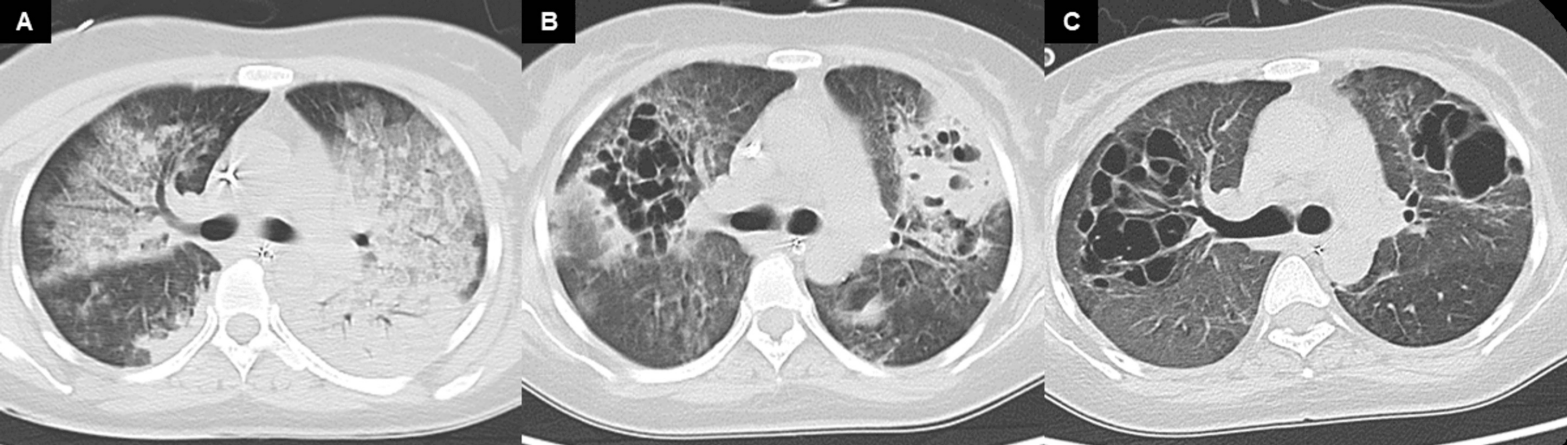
Changes in chest CT findings (A) On admission, CT scans revealed consolidation in the left lower lobe and bilateral GGO. (B) By the time of ECMO weaning, the GGO had resolved, but bilateral cavitary lesions from alveolar destruction and localized consolidation in the left lung were observed. (C) At ICU discharge, the cavitary lesions remained, though the consolidation had resolved. ECMO: extracorporeal membrane oxygenation; GGO: ground-glass opacities
